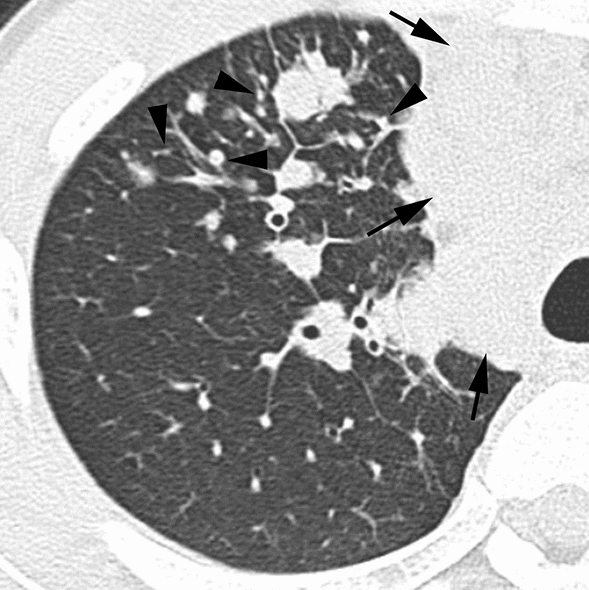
Pulmonary infiltration due to underlying malignancy (lymphoma infiltration) in a 23-year-old male patient with non-Hodgkin lymphoma. HRCT image of the right upper lung showing multiple nodules varying in size (arrowheads), some of which attached to the pulmonary arteries, veins, or ILS, reflecting a perilymphatic distribution. Note the enlargement of the mediastinum with LN enlargement (arrows), which is not clear due to the lung window setting of this CT image
